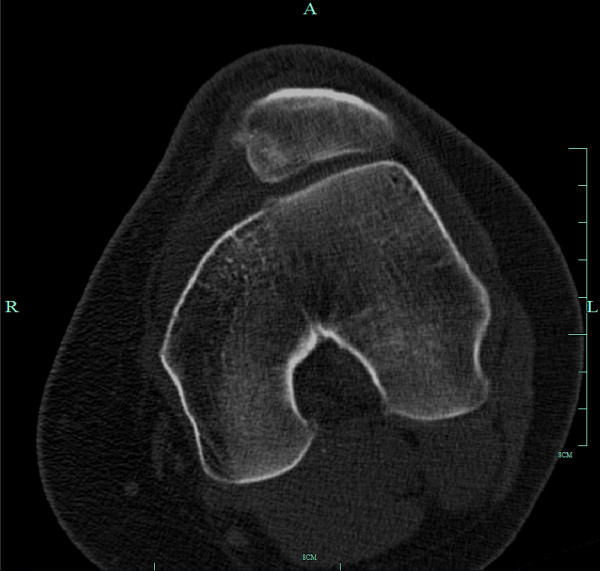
Preoperative axial CT of the left knee showing trochlear dysplasia.
